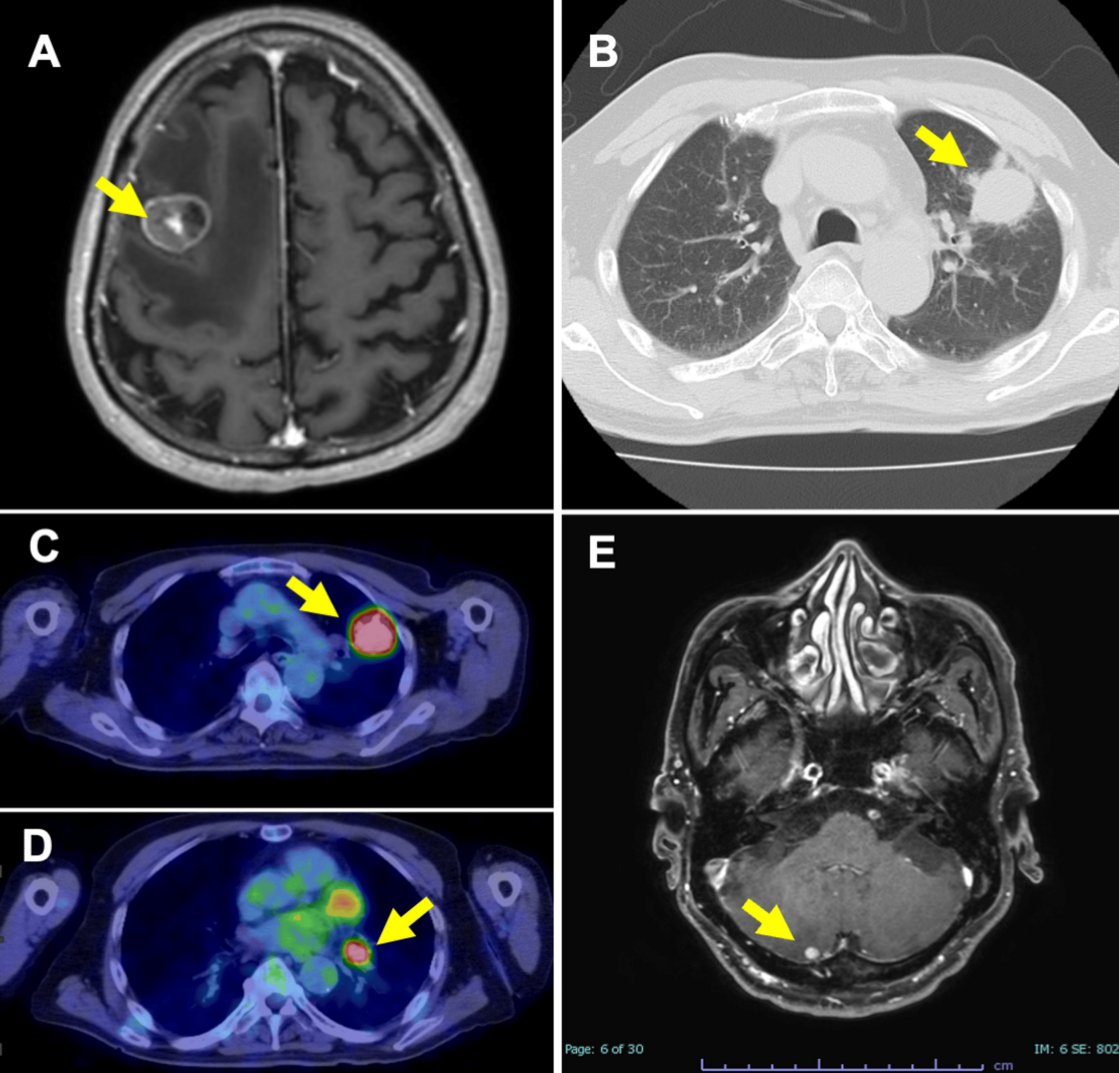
Imaging findings at initial presentation MRI: Magnetic resonance imaging; CT: computed tomography; PET-CT: positron emission tomography-CT (A) Brain MRI (T1-weighted image) showing a 20 mm tumor in the right frontal lobe (arrow). (B) Chest CT revealing a 32 mm mass in the left upper lobe of the lung (arrow). (C, D) PET-CT demonstrating abnormal uptake in the primary lesion and left hilar lymph nodes (arrows). (E) Brain MRI (T1-weighted image) showing a small enhancing nodule, accompanied by surrounding edema in the right cerebellar hemisphere (an arrow)
